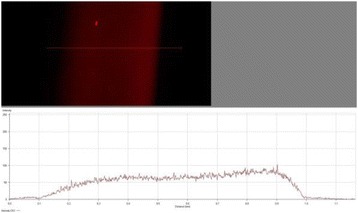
Ultrasound applied. The X­direction is along the ultrasound beam, the Y­axis is the fluorescent intensity. The profile shows the cross­section the sandwich gel
